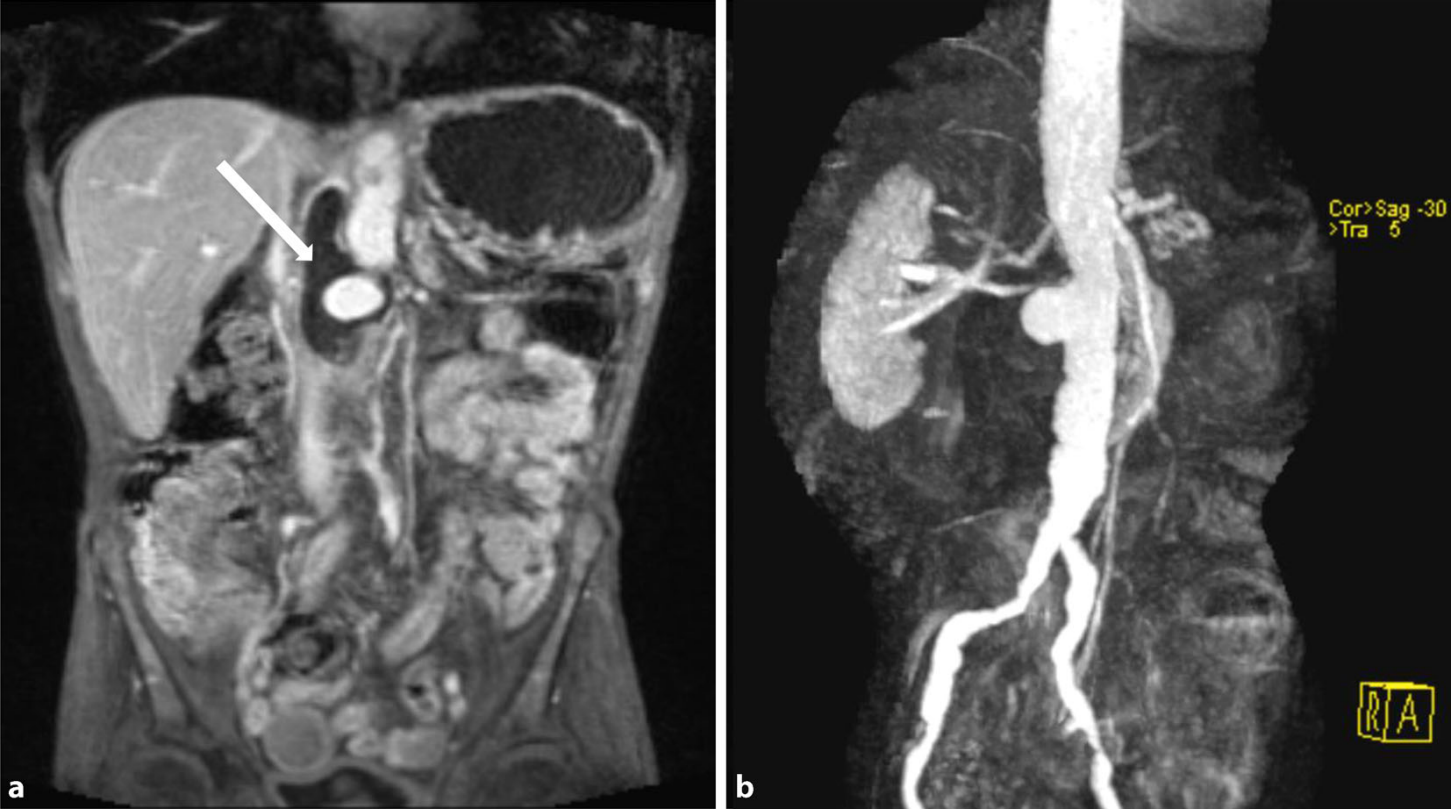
(a) Fast low angle shot (FLASH) magnetic resonance imaging, abdominal (T1 post-contrast medium), coronal view. Partially thrombosed, infectious false aneurysm of the abdominal aorta (*arrow*). (b) Magnetic resonance angiography of the abdominal aorta in the same patient. The extent of the finding as well as its inflammatory cause are distinctly underestimated in the maximum intensity projection (MIP) only reconstruction. Additional axial and coronal sequences are required in the diagnostic work-up for aortitis, in addition to magnetic resonance imaging assessment of morphology
